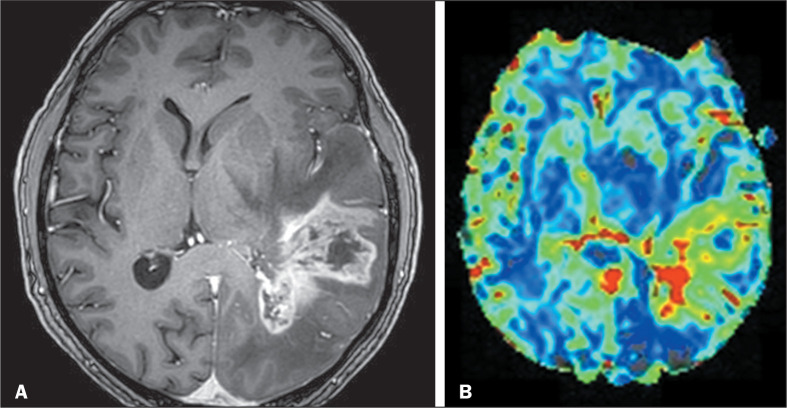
MRI scans of a 63-year-old male patient with a WHO grade 4, IDH-wild
